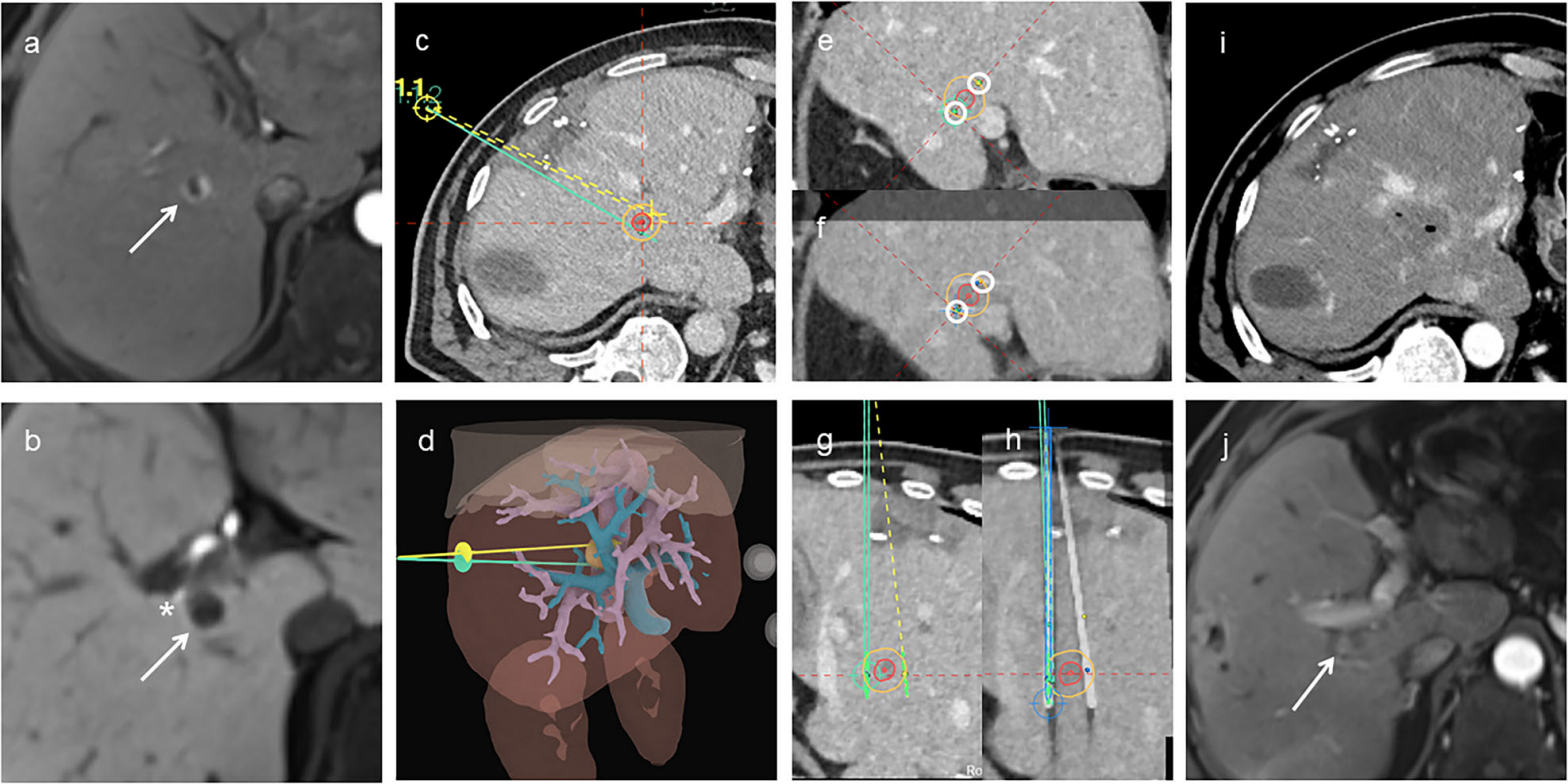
Stereotactic two-needle irreversible electroporation for neuroendocrine liver metastasis near the bile duct. a, b Axial arterial and hepatobiliary phase MRI showing peripherally enhancing tumor (arrow) near second-order bile duct (*). c Axial planning CT with needle trajectories; tumor (red) and 5 mm margin (yellow) outlined. d Three-dimensional liver rendering showing tumor (orange), hepatic veins (purple), portal vein (cyan), and planned needles. e Planning CT orthogonal to the needle axis showing tumor (red), margin (yellow), and planned needle paths (circles). f Fused CT confirming actual needle paths closely matched the plan. g Planning CT in a plane parallel to the needle axis, showing planned trajectories and active tips (green). Due to pleural proximity, a non-parallel orientation was selected. h Fused post-placement and planning CT confirming accurate needle alignment. i Immediate post-procedure CT showing tumor encompassed by the ablation zone. j Three-month MRI showing no residual tumor with parenchymal defect (arrow). CT, Computed tomography; MRI, Magnetic resonance imaging
